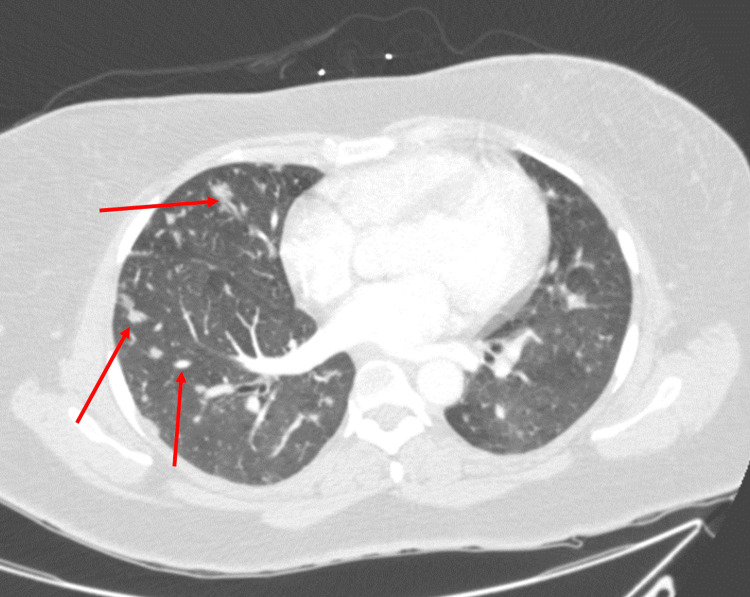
Computed tomography angiography of the chest with contrast demonstrating innumerable sub-centimeter nodules in a perilymphatic distribution.
